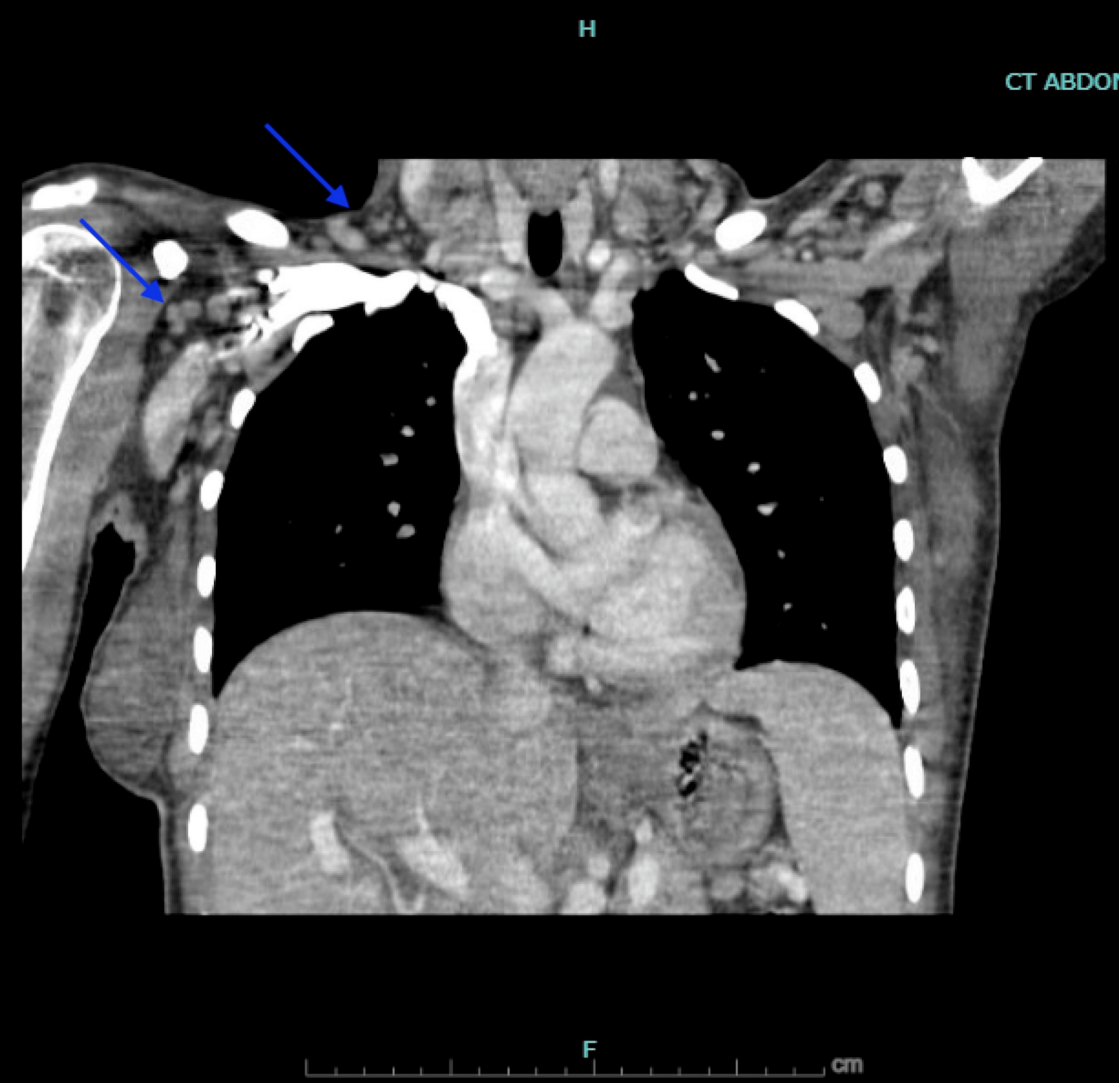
CT Chest with Intravenous Contrast in the Coronal Plane Blue arrows show numerous subcentimeter right axillary and supraclavicular lymph nodes. CT: Computed Tomography
